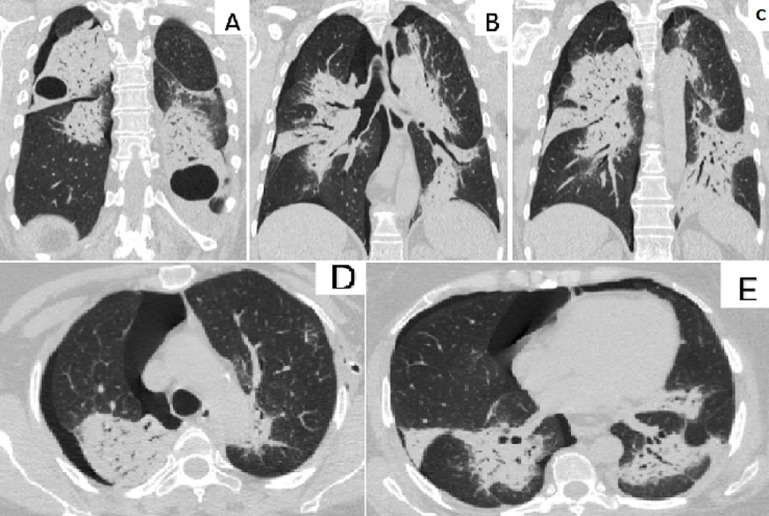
(A+B+C) coronal and (D+E) transverse computed tomography chest scan after bilateral drainage: bilateral pulmonary consolidation associated to bilateral air cysts and bilateral residual pneumothorax
